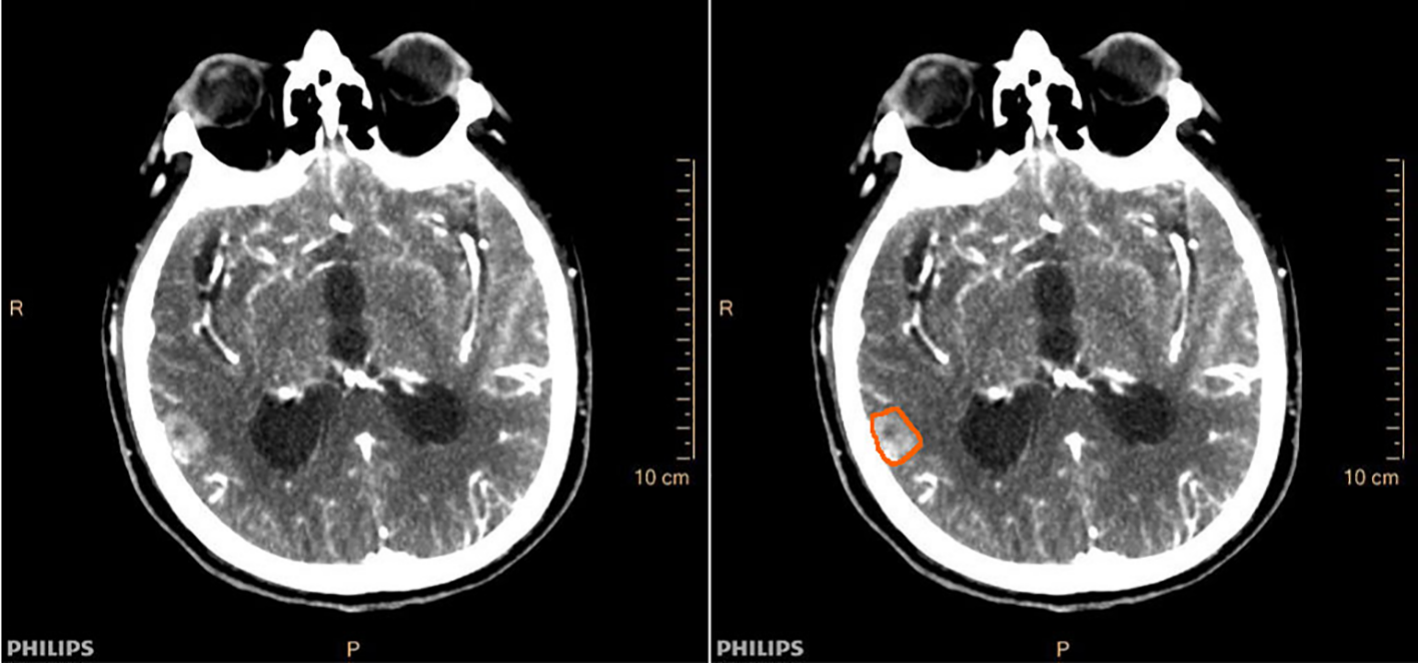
Cranial CT perfusion imaging (left) and region of interest (ROI) outline of intracranial metastases (right) in a patient (male, 78 years old) with brain metastases from lung adenocarcinoma.
